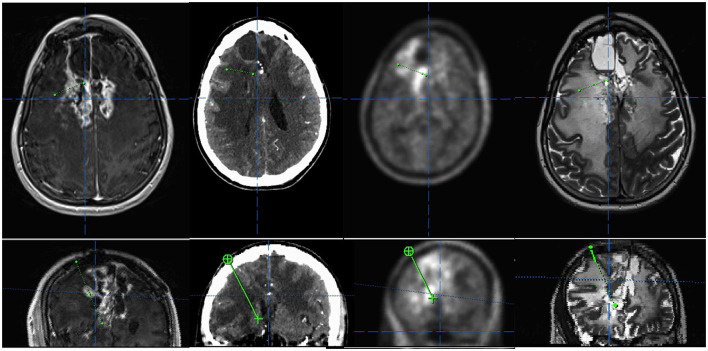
Example of a multimodal trajectory planning targeting both contrast- and (fluorethyl)-L-tyrosine (FET)-enhancing areas in a case of a suspected recurrence of a multimodally treated oligodendroglioma, IDH mutated and 1p/19q co-deleted, the central nervous system (CNS) WHO grade 3. (Upper panel) Axial view of contrast enhanced T1, CT, FET positron emission tomography (PET), and T2. (Lower panel) Inline view depicting the trajectory plane.
